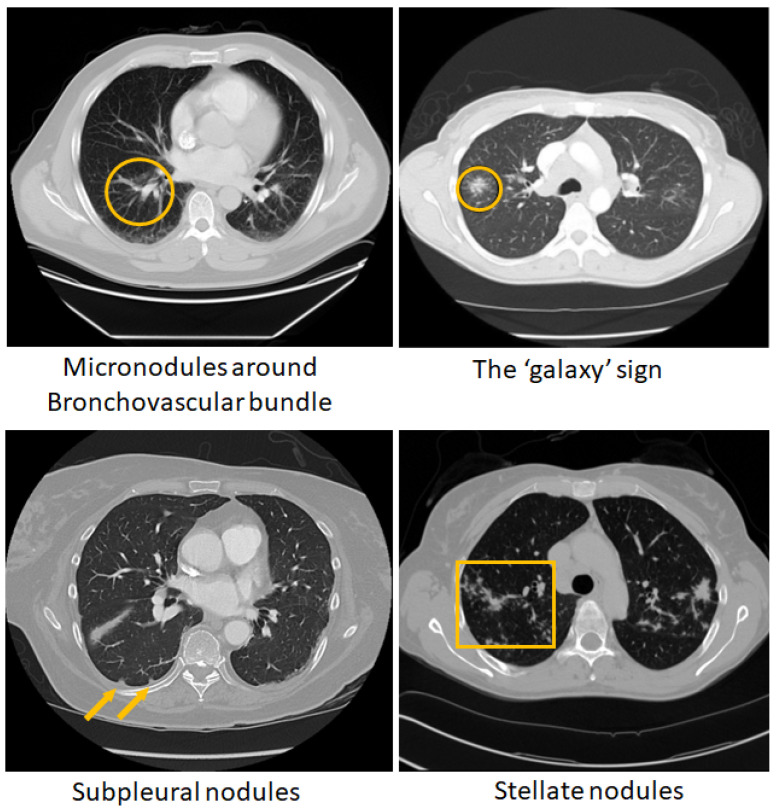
Visible patterns of pulmonary sarcoidosis on chest CT marked in ‘yellow’ circles, arrows and boxes.
